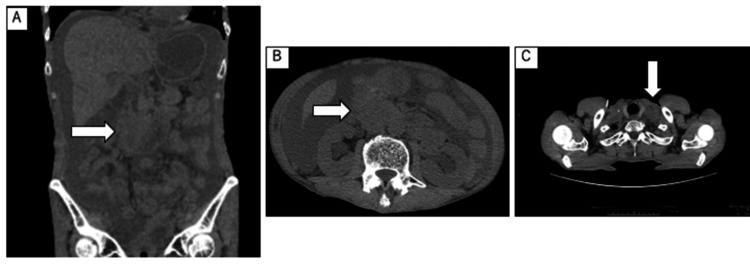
Plain CT scan at admission Plain CT revealing stenosis of the duodenum due to abdominal lymphadenopathy (A, B) and the shrinkage of the left cervical lymph node (C) (white arrows) CT: computed tomography
